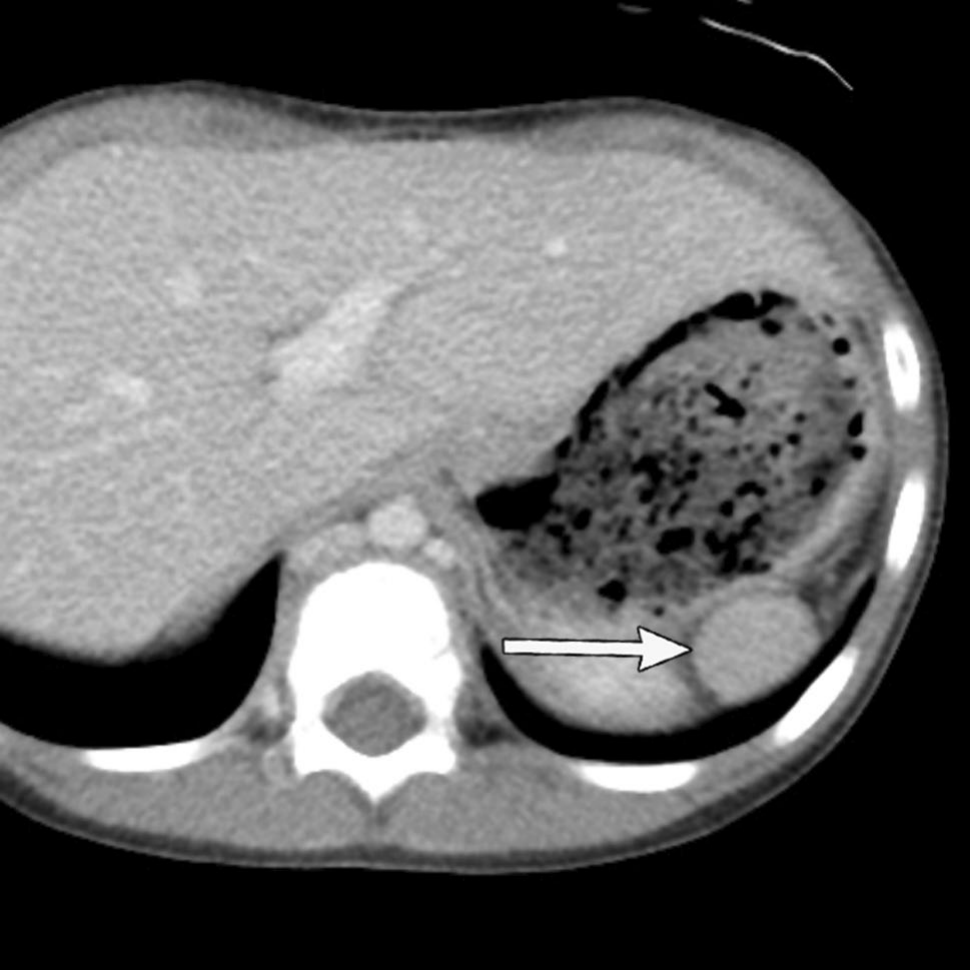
Hyposplenism in a 1-year-old boy with bacterial meningitis. Howell–Jolly bodies and target cells were confirmed. Contrast-enhanced computed tomography imaging confirmed the presence of a small singular spleen without a parent spleen (white arrow)
